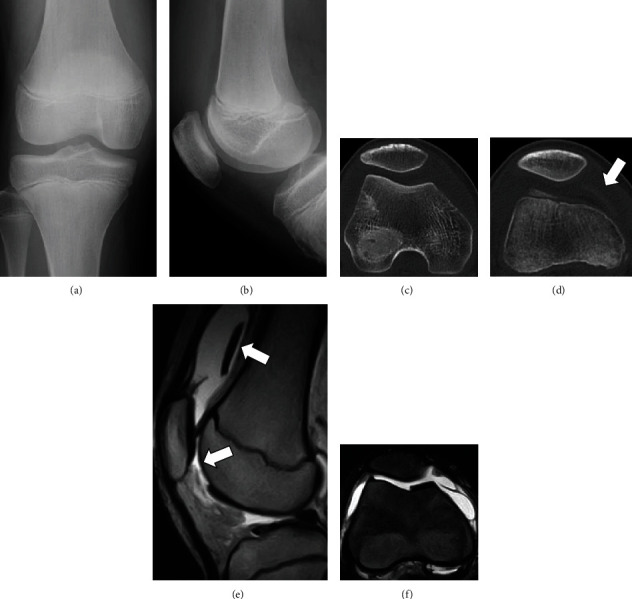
Representative images of case 1. Preoperative anteroposterior (a) and lateral (b) radiography and axial computerized tomography of the knee showing no abnormality (c) and a chondral fragment with no bony element (d). Preoperative sagittal (e) T2-weighted and axial (f) T2-weighted, fat-suppressed magnetic resonance imaging showing a chondral defect in the lateral femoral condyle and a loose chondral fragment in the medial suprapatellar pouch.
